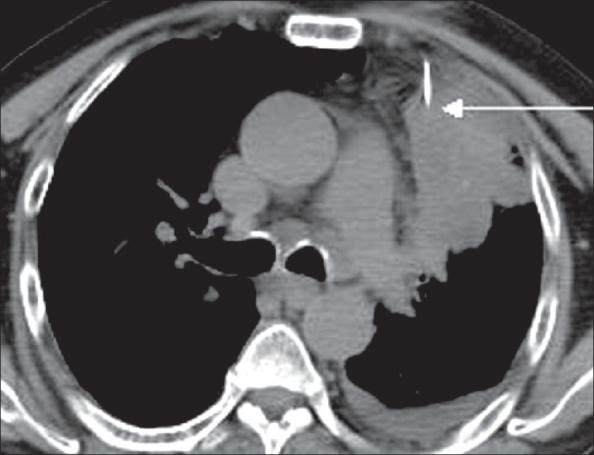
Plain CT of the chest shows the needle in position (arrow) in the area of maximum metabolic activity
